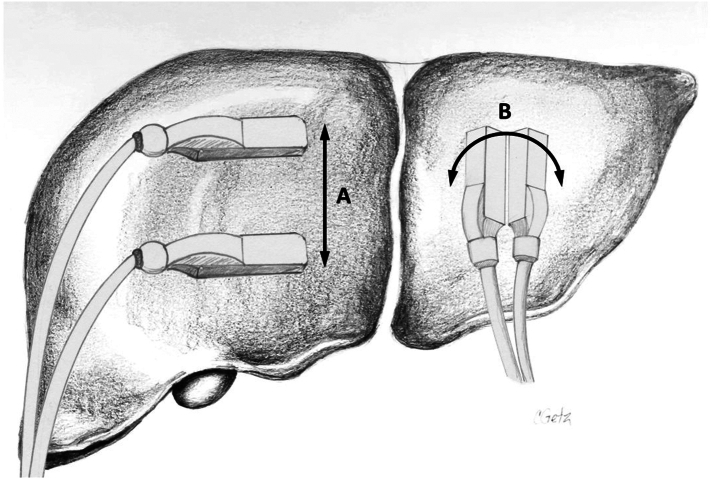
Liver scanning techniques involve (A) sliding and (B) rolling/tilting the ultrasound probe.
